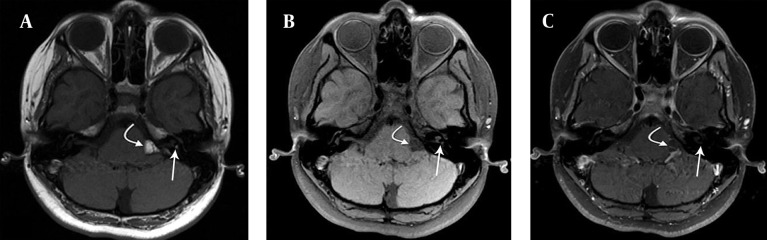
A) Axial T1-weighted image shows a hyperintense mass due to lipoma in the left cerebellopontine angle (curved white arrow) and left internal acoustic canal (straight white arrow). B) Axial T1-weighted image with fat saturation displays saturation of lipomas in the left cerebellopontine angle (curved white arrow) and left vestibule (straight white arrow). C) Enhanced axial fat saturation T1-weighted MR image shows no enhancement of lesions
